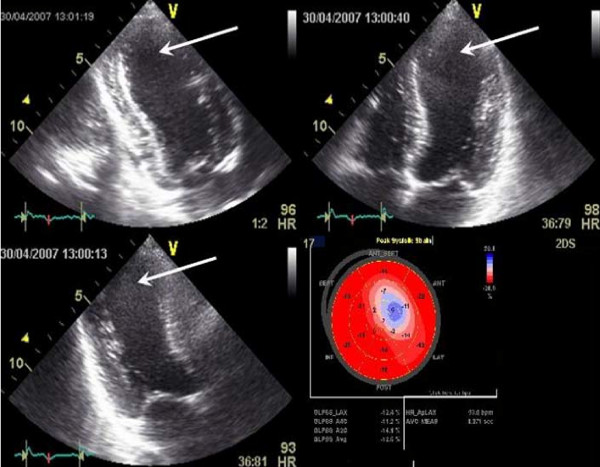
Echocardiographic image showing left ventricular wall motion abnormalities of the apex in systole (arrows): apical two chamber view (left upper panel), four chamber view (right upper panel) and three chamber view (left lower panel). Matching bulls eye display of left ventricular segments strain quantitative analysis (right lower panel). Reduced myocardial contraction within the region of myocardial infarction is clearly visible (blue and pink colour).
